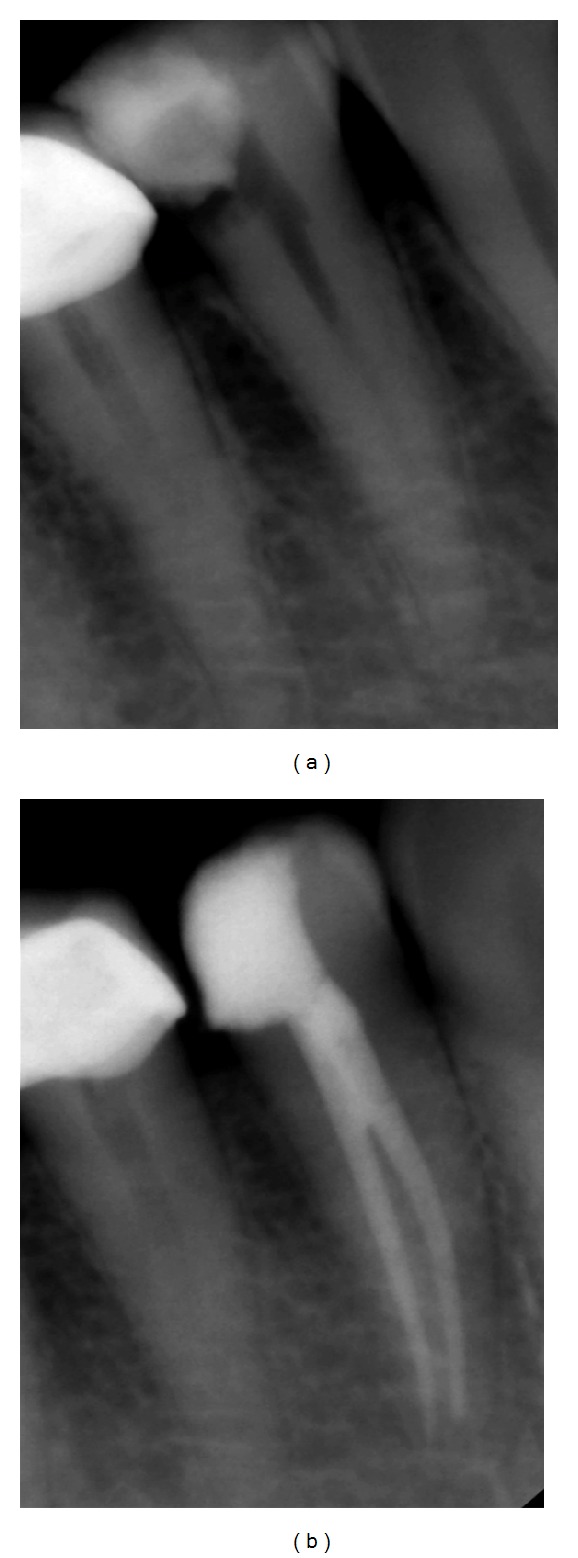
(a) A sudden change in radiographic density at the coronal third indicates canal bifurcation, which is distinctly visible on an angulated radiograph (b).
